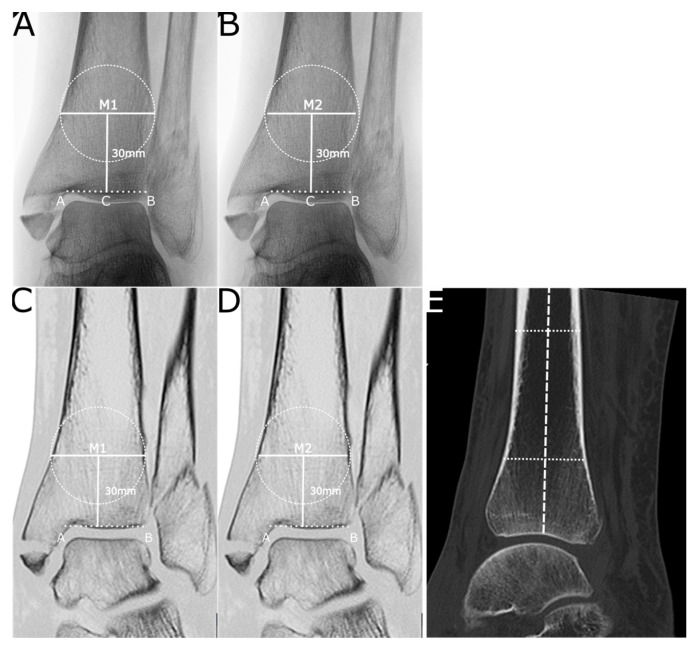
(A) AP radiograph showing the method of measurement of the cortical bone thickness at 30 mm proximal to the tibial joint line according to Patterson et al. [11]. The cortical bone thickness was calculated as the difference of M2 and M1 at 30 mm (R1) and 50 mm (R2). (B) The same method was applied in coronal CT images of the same ankle joint at 30 mm and 50 mm (C,D). In the sagittal view (E), the middle of the tibia was determined, and the corresponding coronal view used to measure the cortical bone thickness. The layer thickness of the CT images was at least 3 mm.
